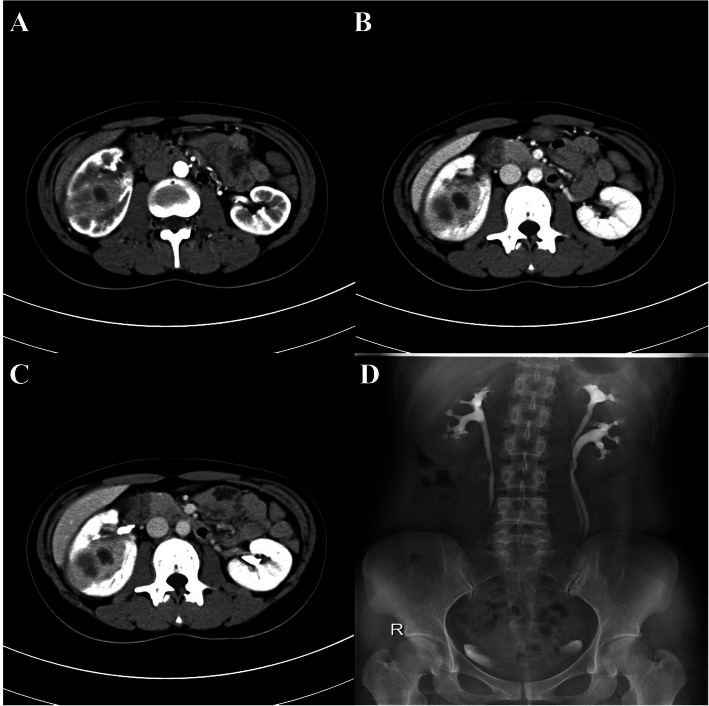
Contrast-enhanced CT scan showing a 6.0 cm × 4.9 cm heterogeneous enhancing mass with hypodense areas within it in the middle of right kidney (a, b and c). Intravenous pyelogram showing absence of dilatation or filling defects in the right renal calyces or pelvis (d)
